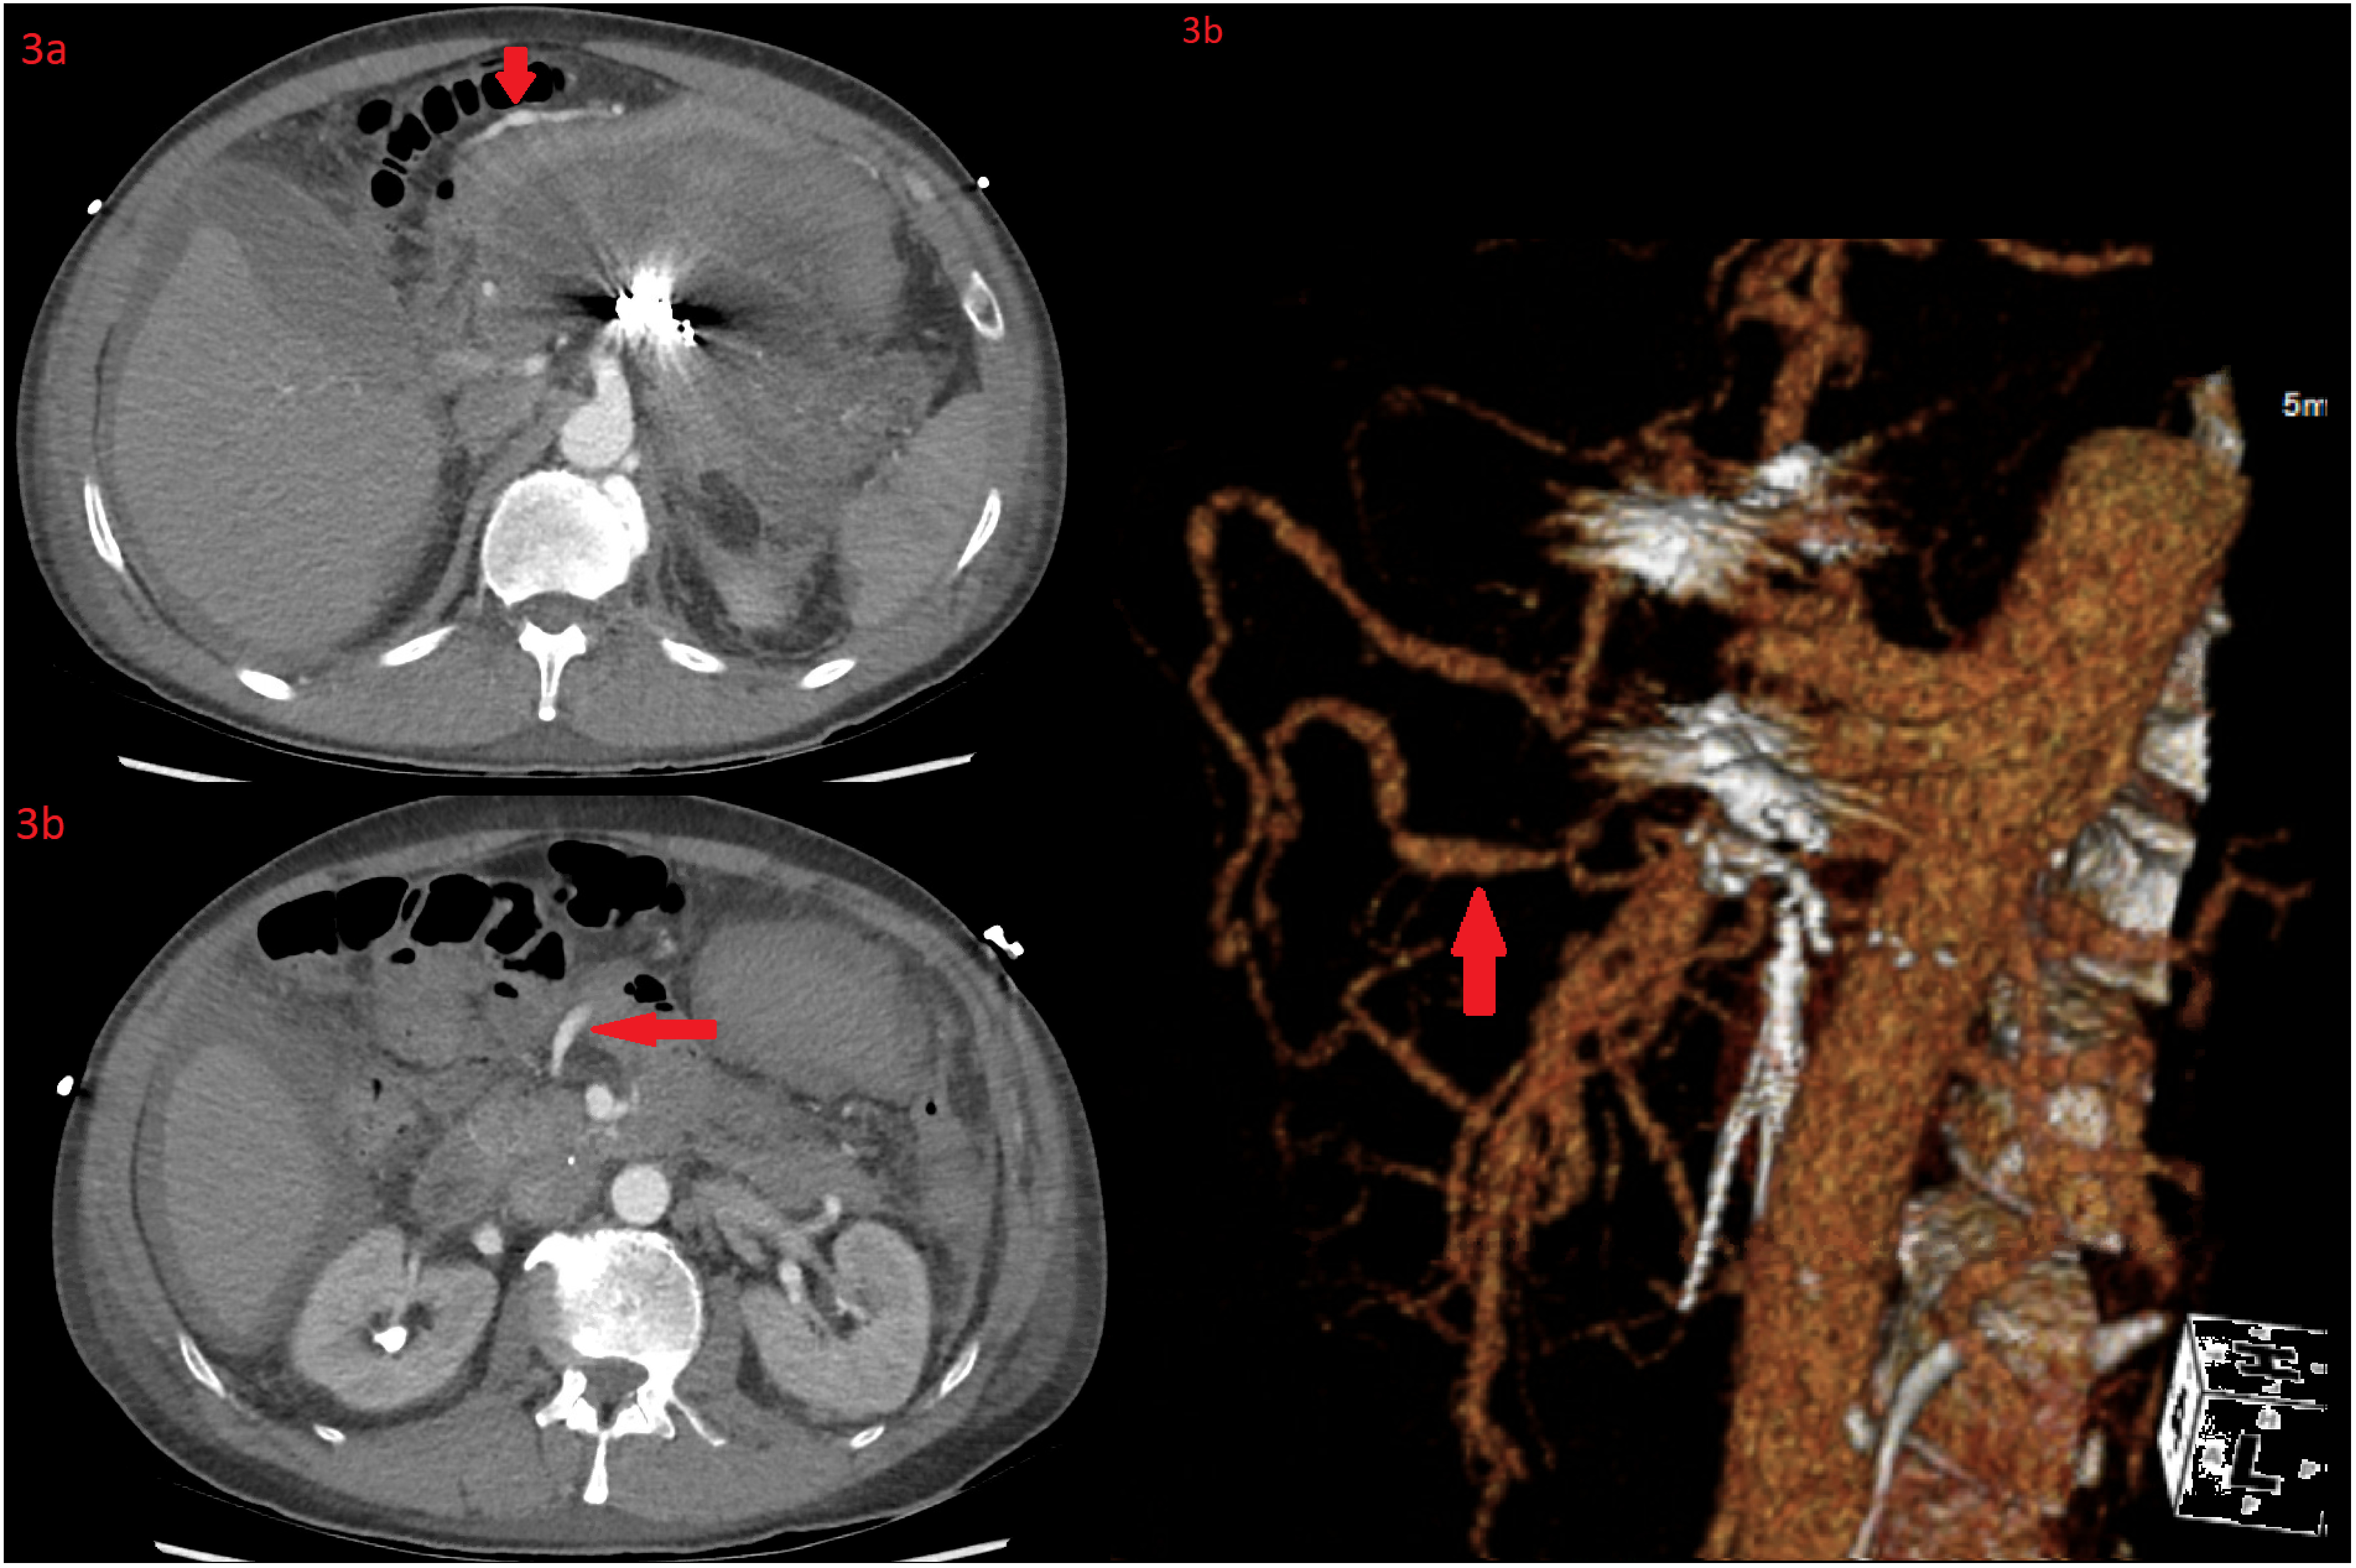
Fig. 3 (a) “String of beads” radiographic findings seen at the gastroepiploic artery. (b) Mid superior mesenteric artery dilatation on computed tomography. (c) 3-Dimensional reconstruction of the mid superior mesenteric artery dilatation.
